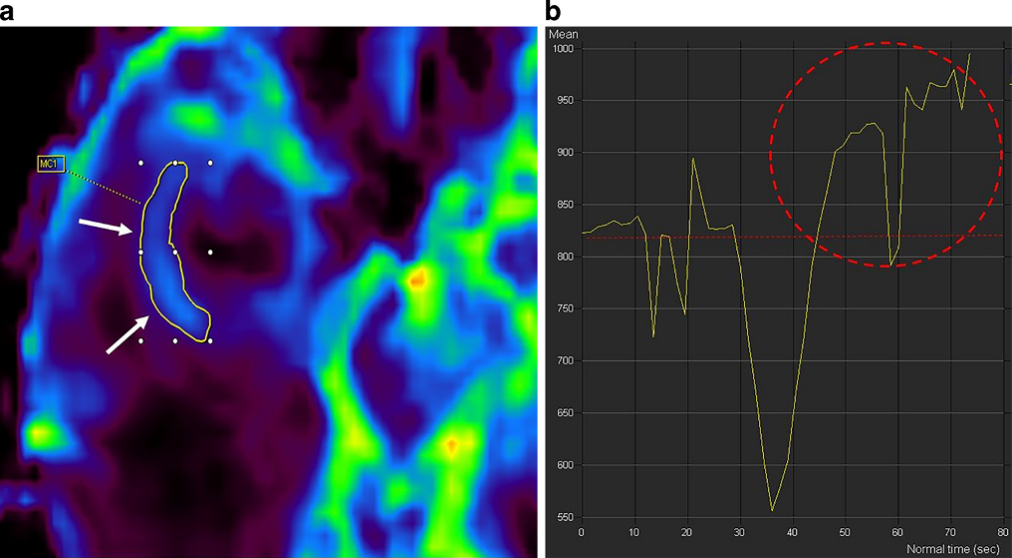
On dynamic susceptibility-weighted, contrast-enhanced (DSC) perfusion MRI the lesion showed increased perfusion on the cerebral blood volume (CBV) map in axial orientation (a, *arrows*). The signal intensity-time curve (b), derived from a region of interest within the CBV map, markedly exceeded the baseline after the first pass (b, *red circle*), a finding considered characteristic for primary cerebral lymphomas [14]
